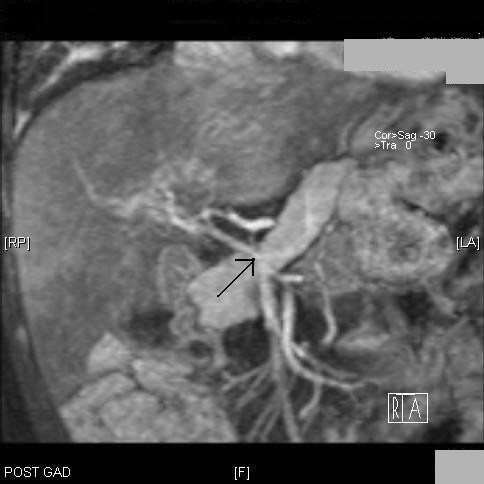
Thready portal vein with prominent collaterals seen in the coronal section of the gadolinium-enhanced MRI scan.
